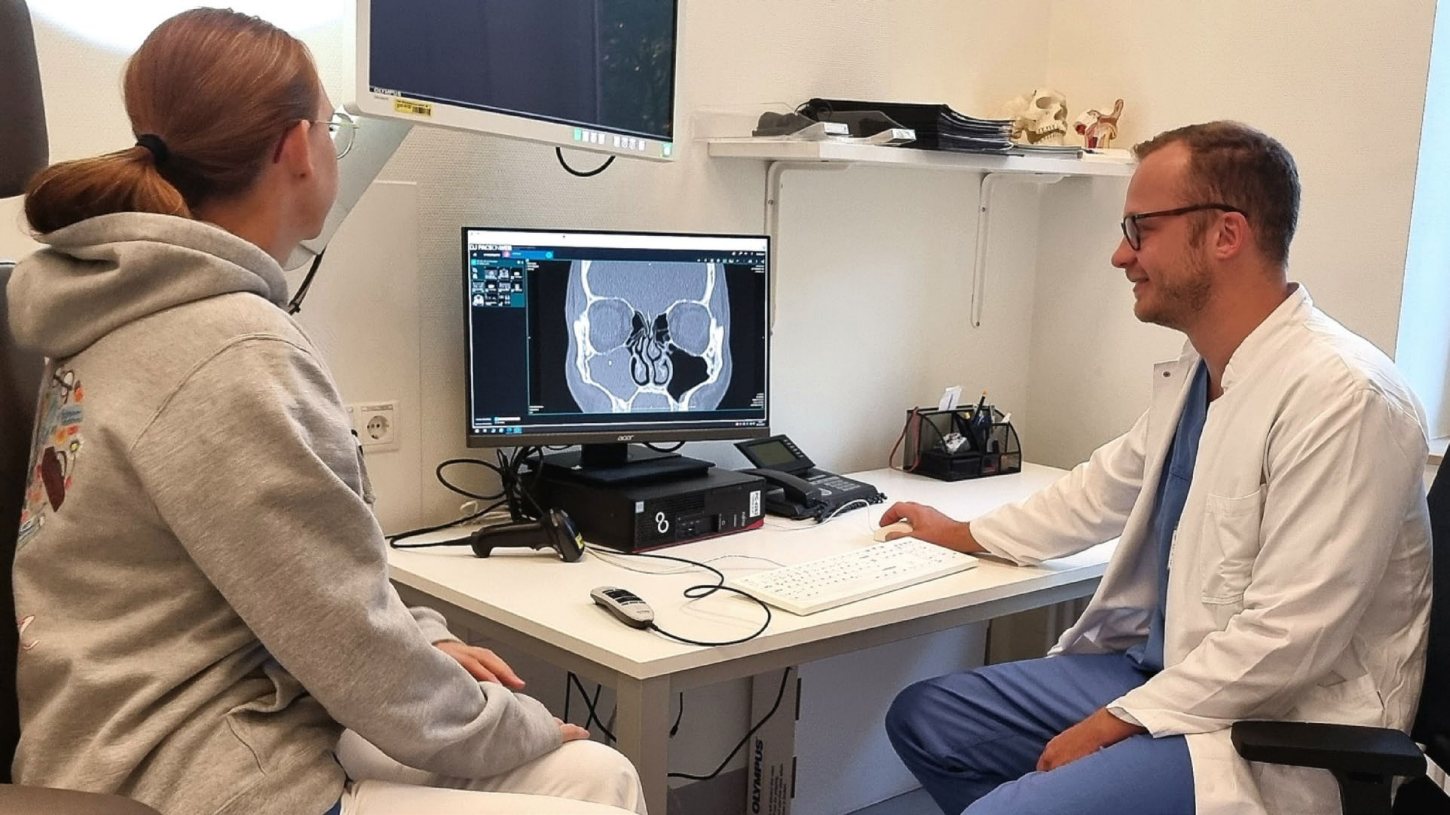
Real educational situation using 2D CT images on a PC screen. Written informed consent was obtained by the participant to publish the image.
